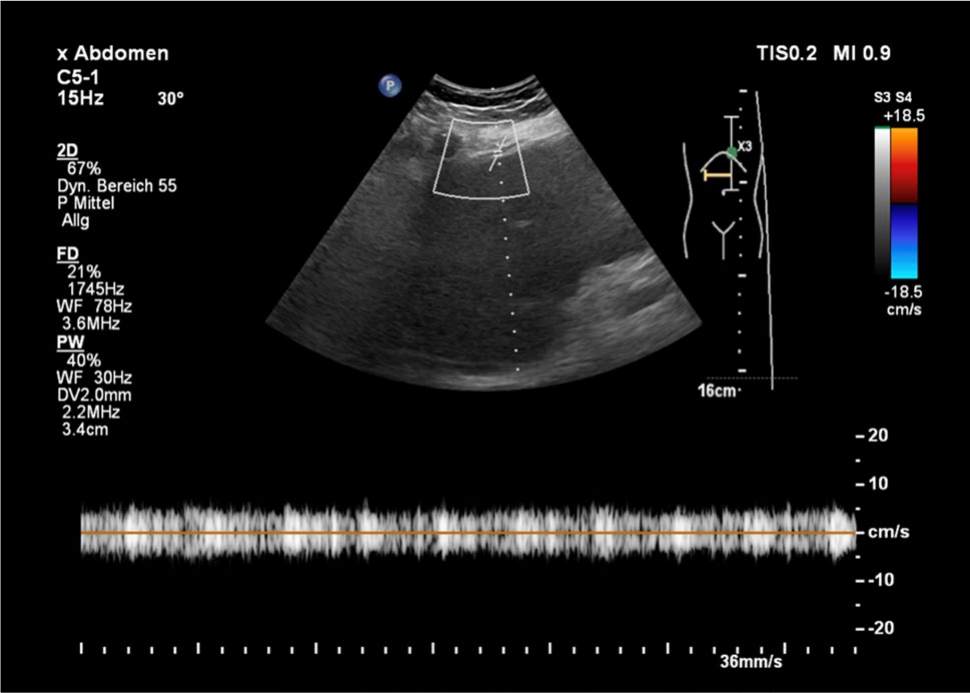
Postoperative Doppler ultrasound showing hepatofugal flow in the round ligament
